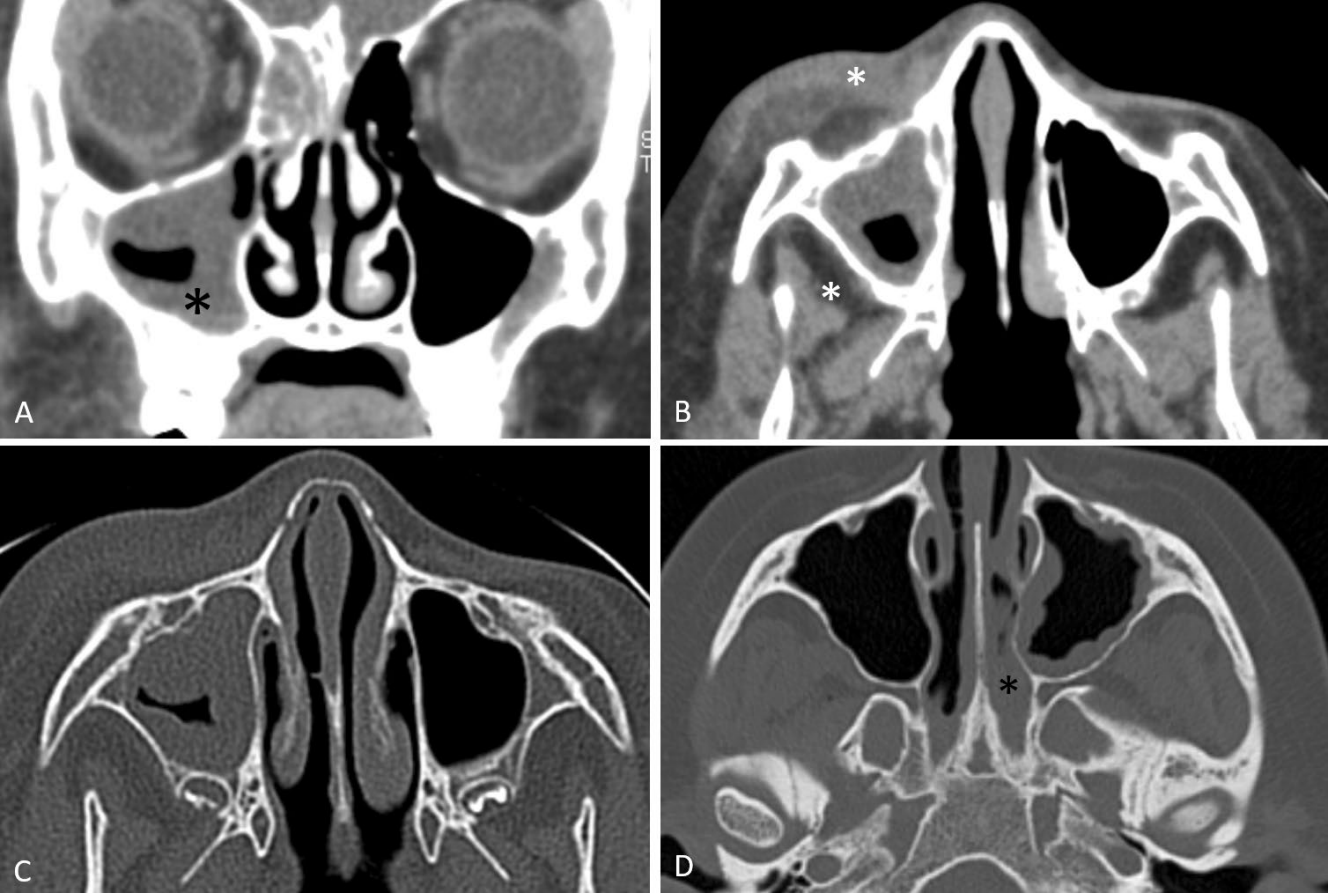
Examples of findings in patients with *Aspergillus* AIFR. (A) Coronal CT image shows unilateral mucosal thickening of the right maxillary sinus (asterisk) and the ethmoid air cells. (B, C) Axial images from the same patient shows fatty infiltration of the anterior periantral fat and the posterior periantral fat, without bony dehiscence. (D) Another patient with Aspergillus AIFR with unilateral mucosal thickening of the maxillary sinus and the nasal cavity (asterisk).
